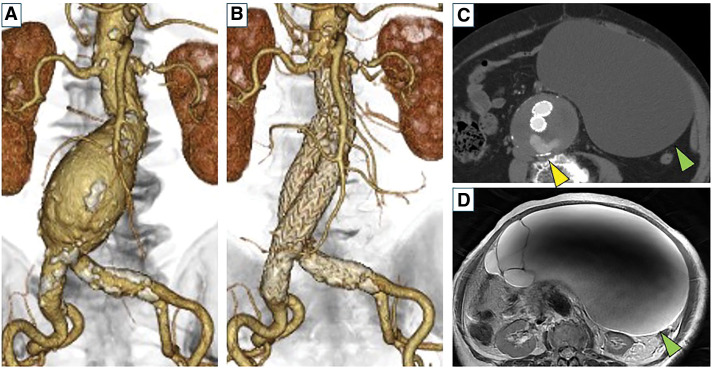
CT radiographs acquired prior to surgery. (A) Contrast-enhanced CT radiograph acquired before EVAR was conducted 7 years ago. A fusiform-shaped abdominal aortic aneurysm was visible below the renal arteries. The inferior mesenteric artery was occluded before EVAR. (B) Contrast-enhanced CT radiograph acquired one week after EVAR. EVAR was performed using Excluder C3 (W. L. Gore & Associates, Flagstaff, AZ, USA), in accordance with the manufacturer’s instructions for its use. The preoperative diameter of the abdominal aortic aneurysm was 50 × 50 mm. (C) Axial image acquired via contrast-enhanced CT one year ago and before coil embolization, showing type II endoleak from the lumbar artery (yellow arrowhead). The abdominal aortic aneurysmal sac had expanded to 62 × 74 mm. A giant ovarian tumor was found in the abdominal cavity (green arrowhead). The volume of the ovarian tumor at the time of imaging was 2395 cm^3^. (D) T2-weighted radiographs acquired via abdominal MRI showing a hyperintense cystic lesion measuring 25 × 21 × 15 cm in the abdominal cavity. There was no internal solid component or wall thickening (green arrowhead), and no obvious malignant findings were observed. EVAR, endovascular aortic repair
